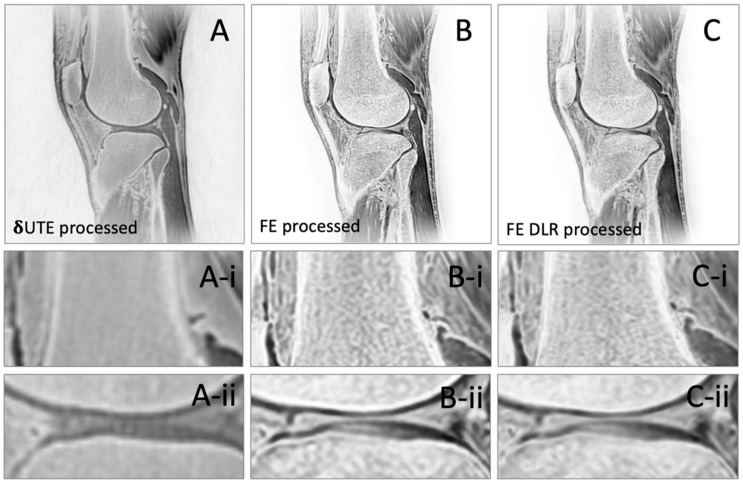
Representative processed images with CT-like contrast, created from (A) δUTE, (B) FE, and (C) FE HR–DLR source images. Images below show magnified sections of (i) cortical bone and (ii) tibiofemoral contact region.
